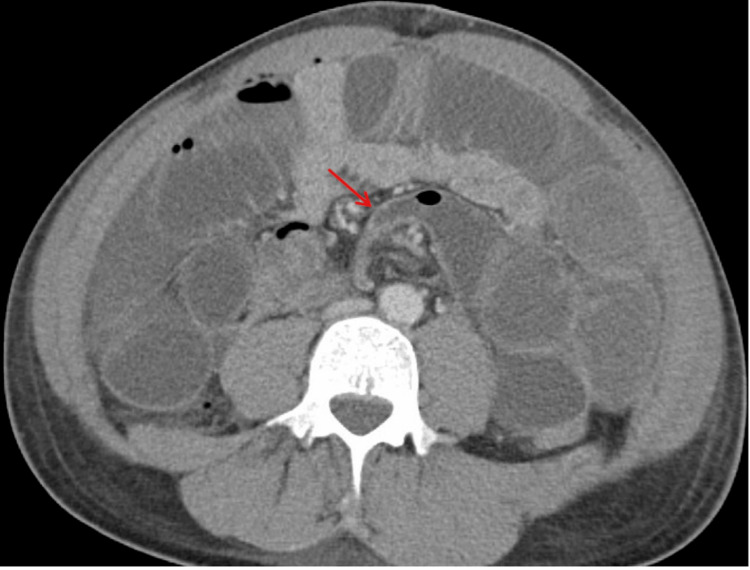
CT abdomen and pelvis with contrast (axial view) with evidence of a small bowel closed loop obstruction (red arrow) seen in the central portion of the abdomen in the mesentery, proximal to the surgical anastomosis.
